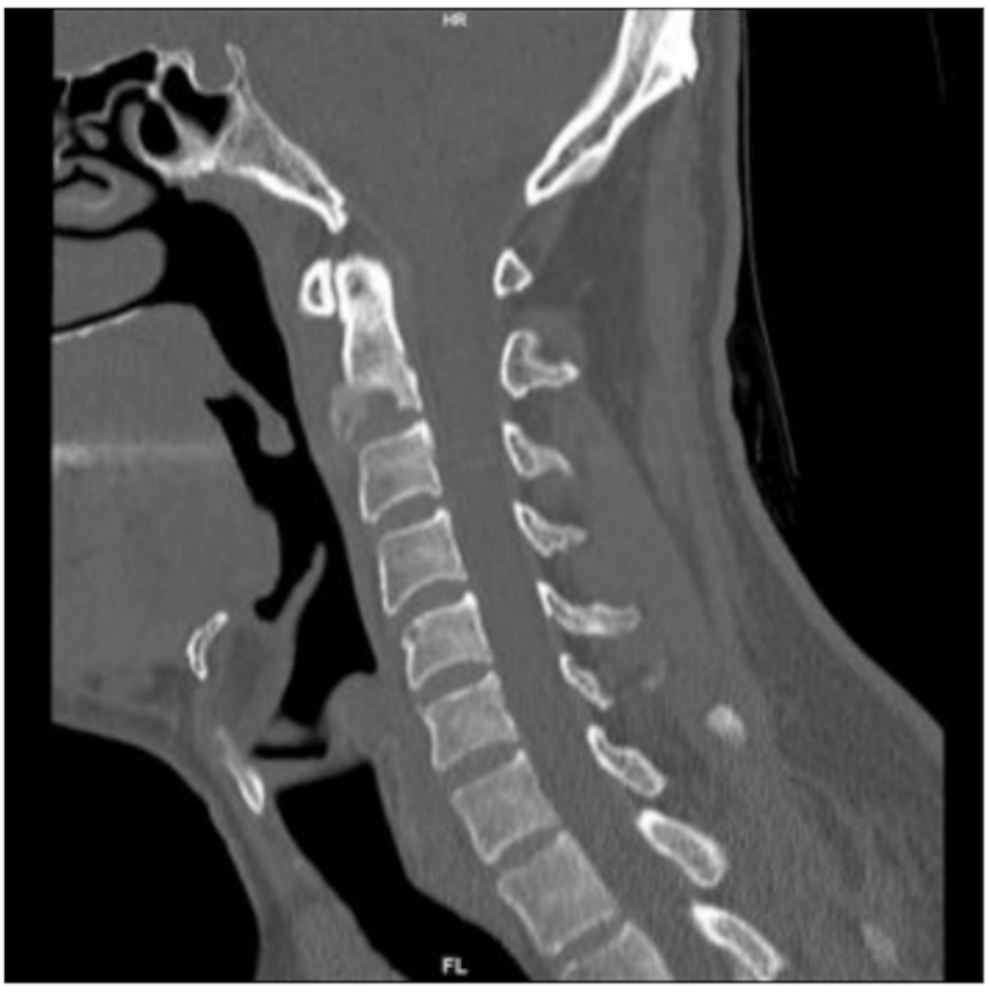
CT scan after 6 weeks showing consolidation of C2 vertebral body and a regression of the prevertebral soft tissue swelling
